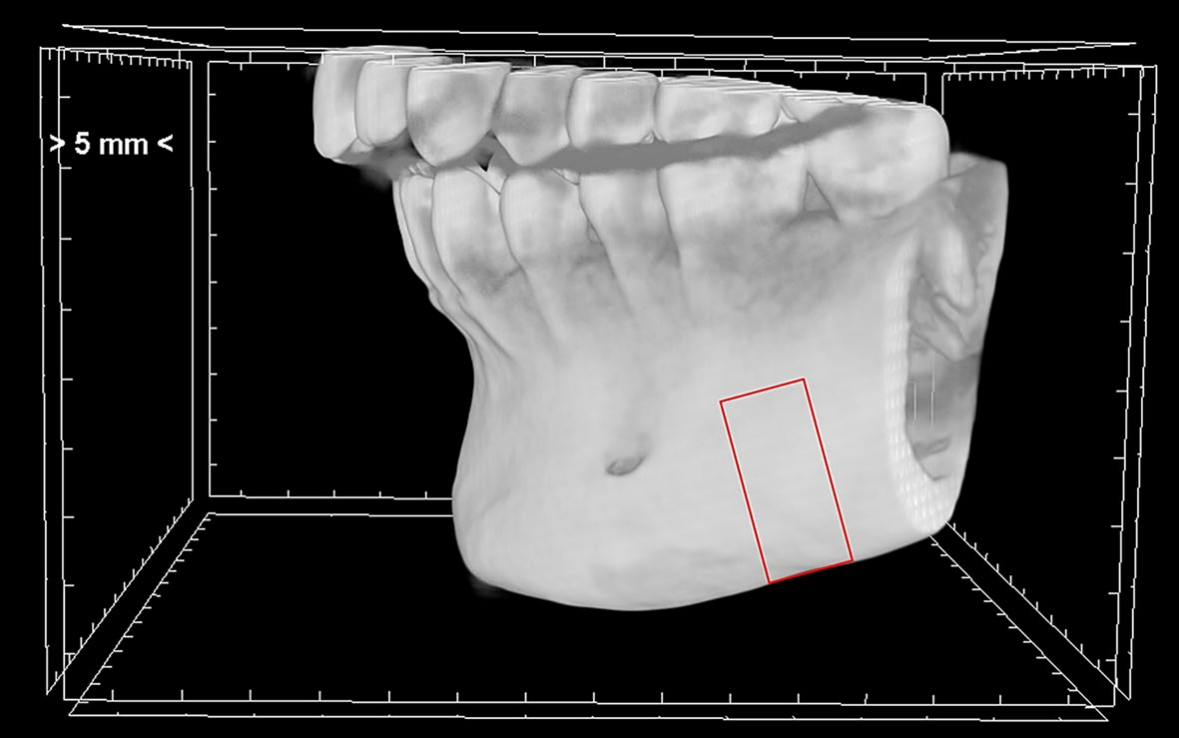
3-Dimensional cone beam reconstruction of lateral view of the mandible depicting the region of interest boundaries
